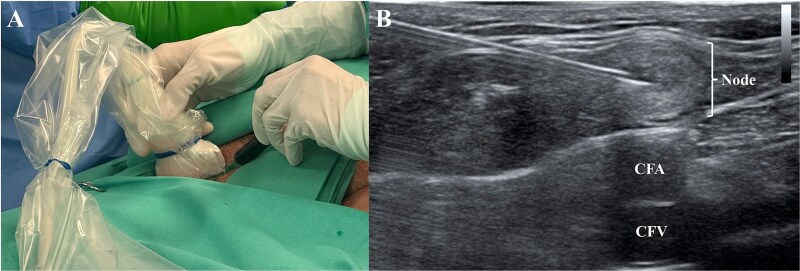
At the same time the cervical reexploration was being performed, the right inguinal area was prepared and under ultrasonographic guidance (A) using a 21G needle, an inguinal lymph node (B) was punctured and the indocyanine green was administered (0.2 mg/kg). CFA: Common femoral artery; CFV: Common femoral vein.
